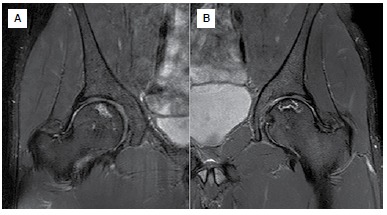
MRI of the hips showing bilateral involvement without joint collapse: (A) right, (B) left.
